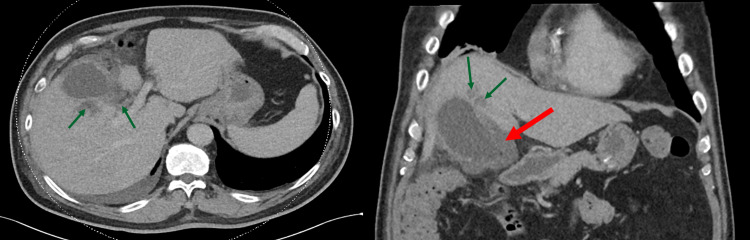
Transverse and coronal CT images of Case 1 showing acute cholecystitis with sealed perforation (red arrow) and adjacent liver abscesses (green arrows). The elevated right hemidiaphragm is also visible on coronal view.
